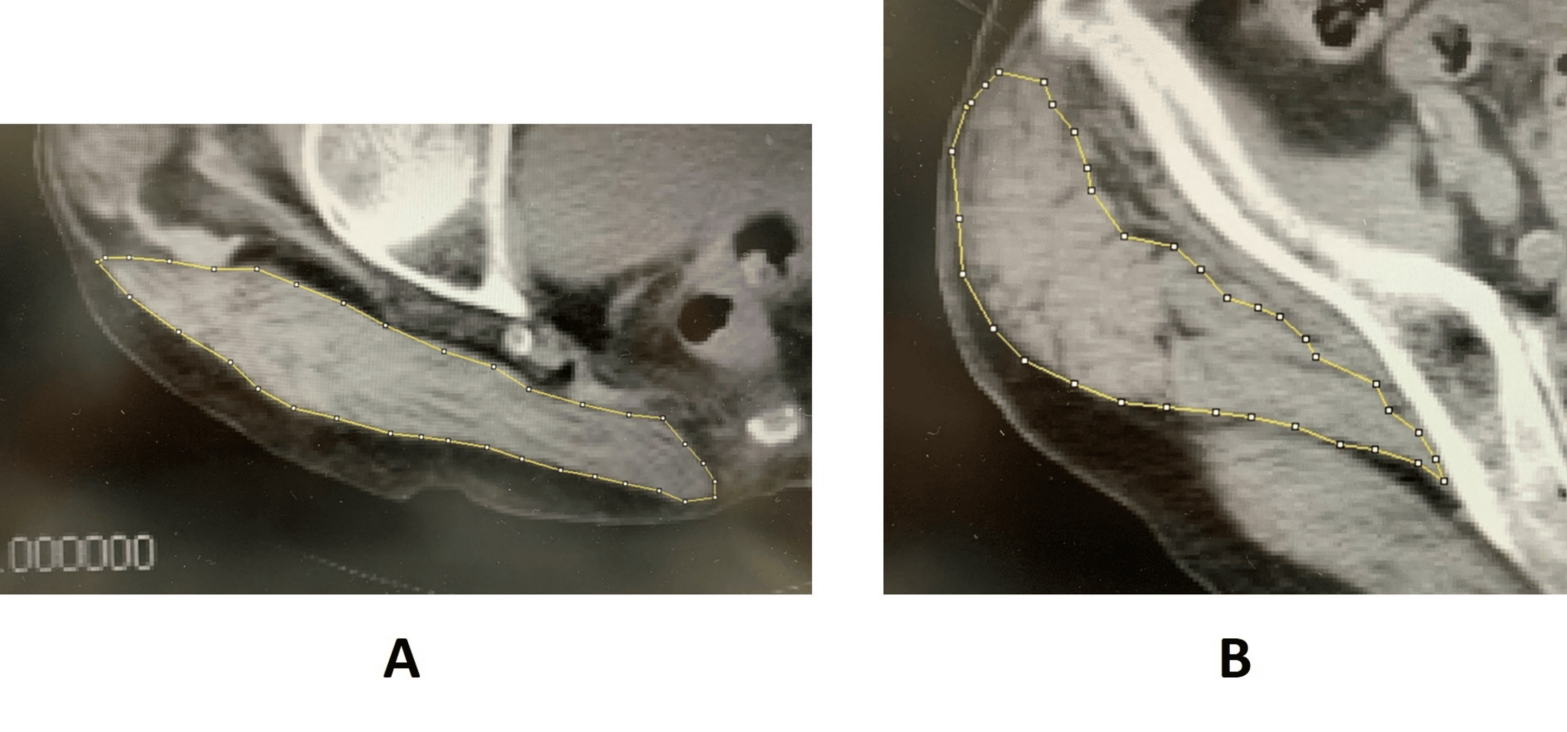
CT images of the gluteus maximus and gluteus medius Axial images at the level of the tip of the greater trochanter represent the gluteus maximus (A), while those at the level of the third sacral vertebra represent the gluteus medius (B).
